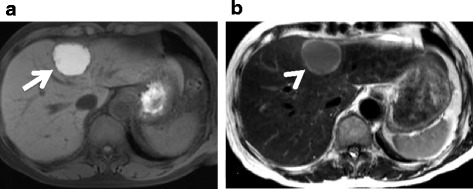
Magnetic resonance imaging revealed a high-intensity tumor on T1-weighted images (a; *arrow*) and a low-intensity tumor with surrounding high intensity on T2-weighted images (b; *arrowhead*)
